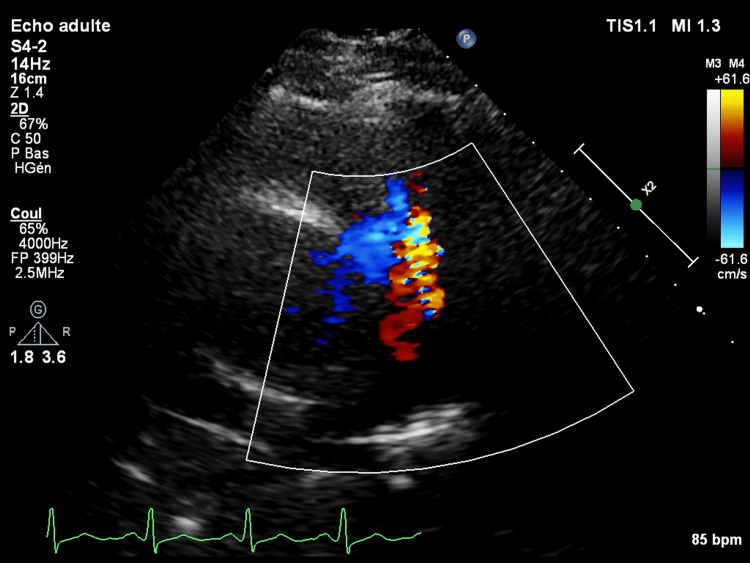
PSAX focus on pulmonary artery showing a PDA with left-to-right shunt PSAX: parasternal short-axis; PDA: patent ductus arteriosus.
